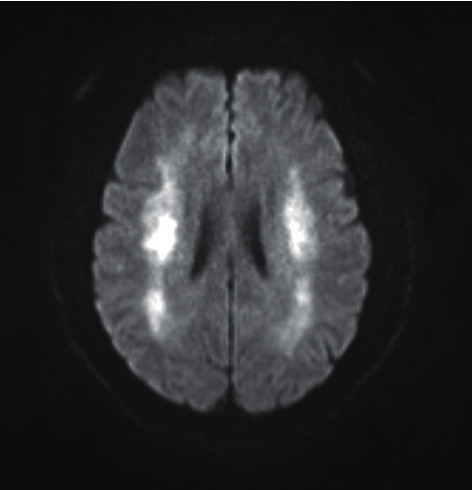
Magnetic resonance imaging findings demonstrating symmetrical bilateral supratentorial restriction in keeping with leukoencephalopathy.
